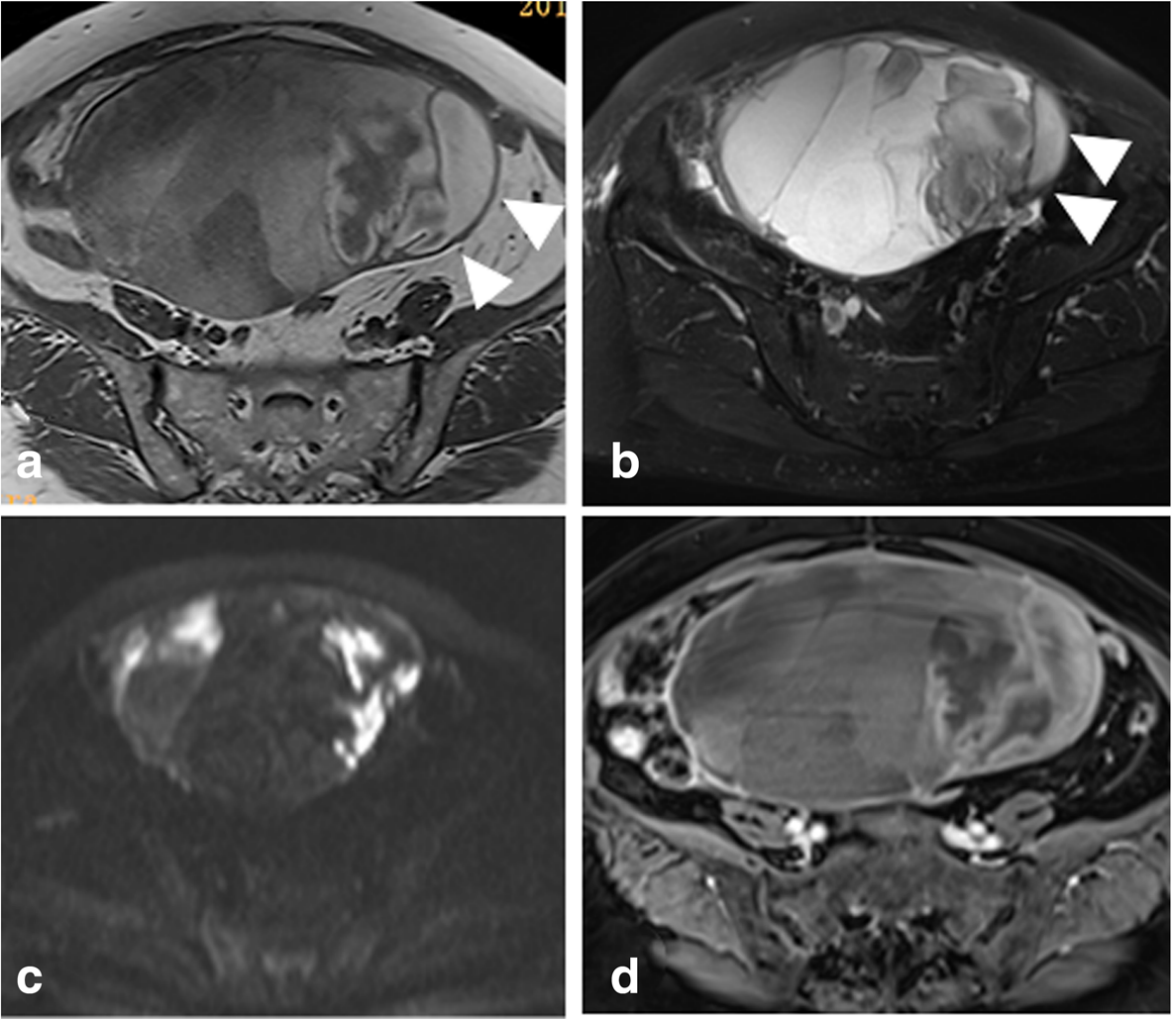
A 54 -year-old woman with primary adult type OGCT (Ic). The mass shows as the purely cystic lesion with mostly high signal on T1WI (a) and T2WI (b). Note, the hemorrhagic contents locates on the left side of the tumor, representing the relatively high signal on T1WI and low signal on T2WI (arrowhead) and high signal on DWI (c). After injection of contrast medium, the cystic wall shows minor enhancement (d)
